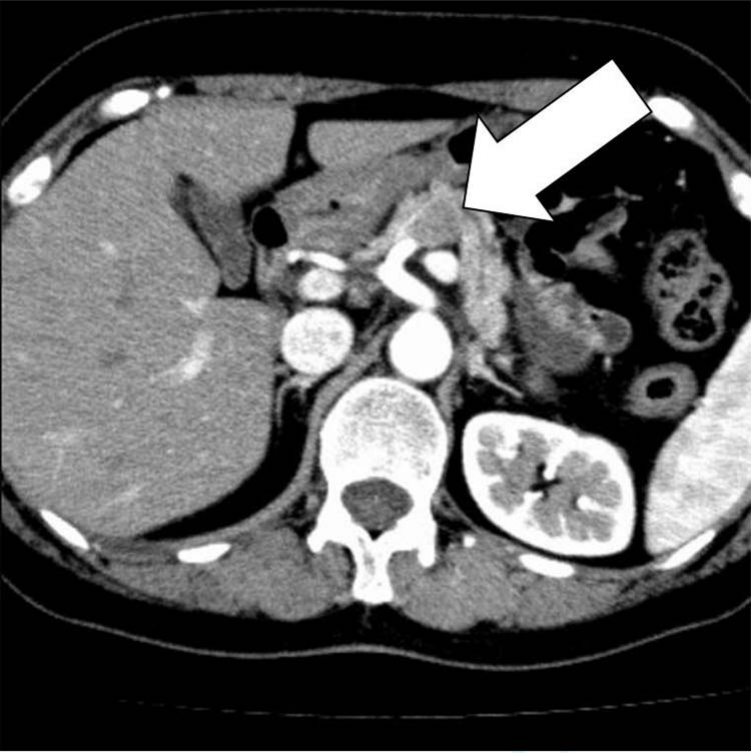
An abdominal CT scan revealed a 2 cm mass with mild contrast effect in the pancreatic body. There was a little tendency to invade the surrounding area, which was different from typical pancreatic ductal carcinoma.
